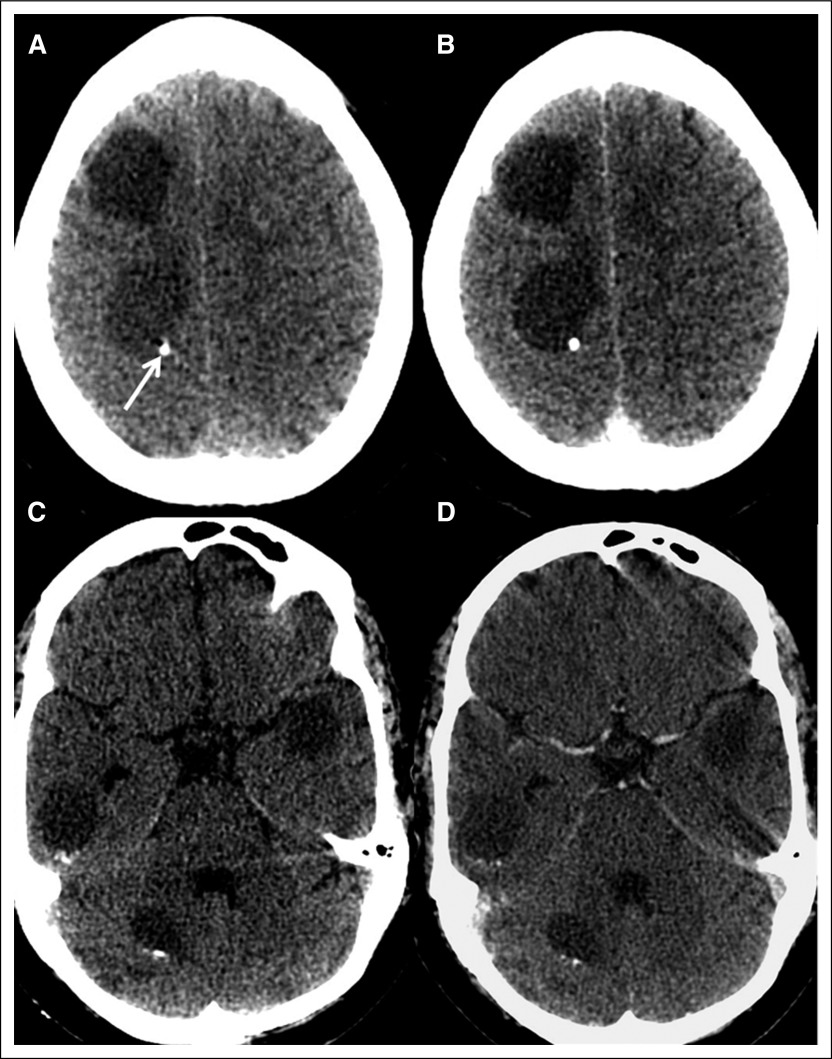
(A and C) Plain and (B and D) postcontrast computed tomography images showing multiple nonenhancing hypodense lesions in the bilateral cerebral and cerebellar hemispheres. These lesions were cystic (nonenhancing hypodense areas), and a few of them showed eccentric calcification (hyperdense foci; arrow).
